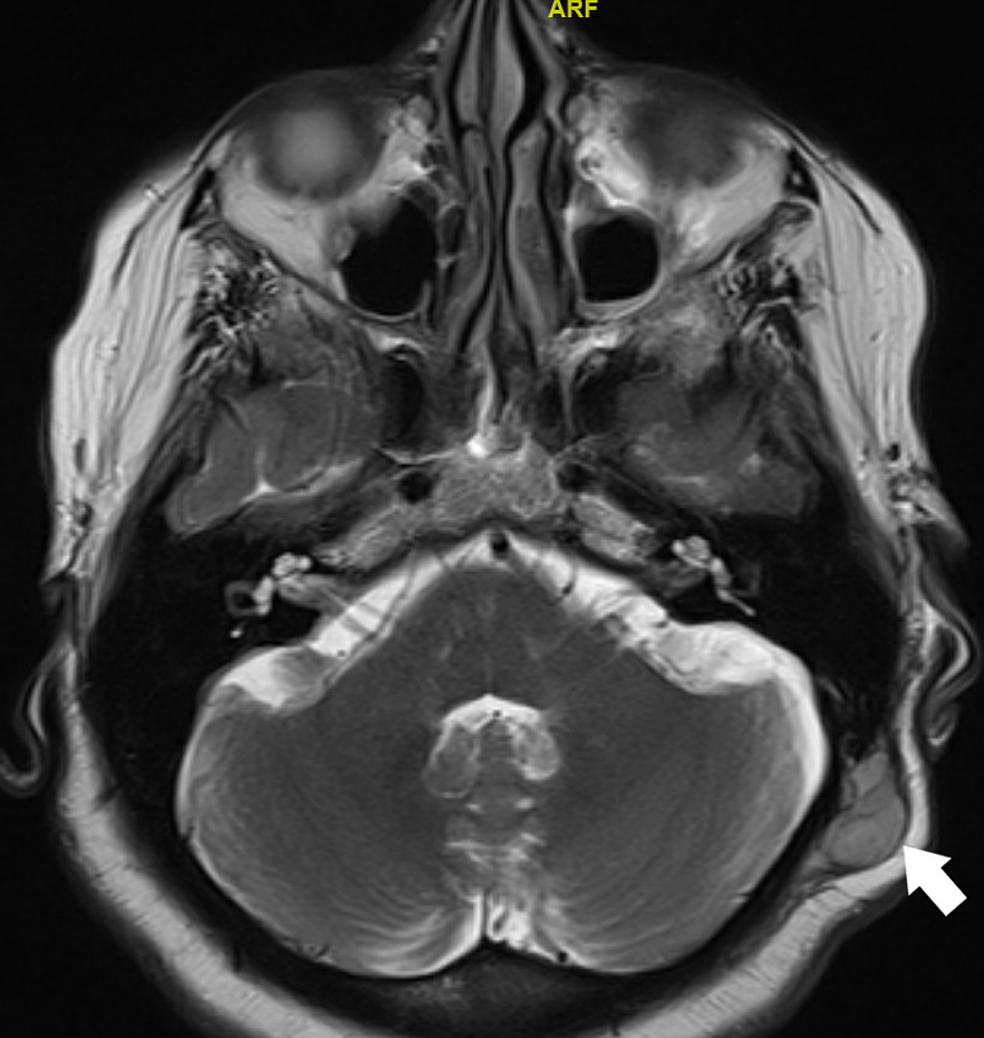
MRI showing the lower lesion (white arrow).
